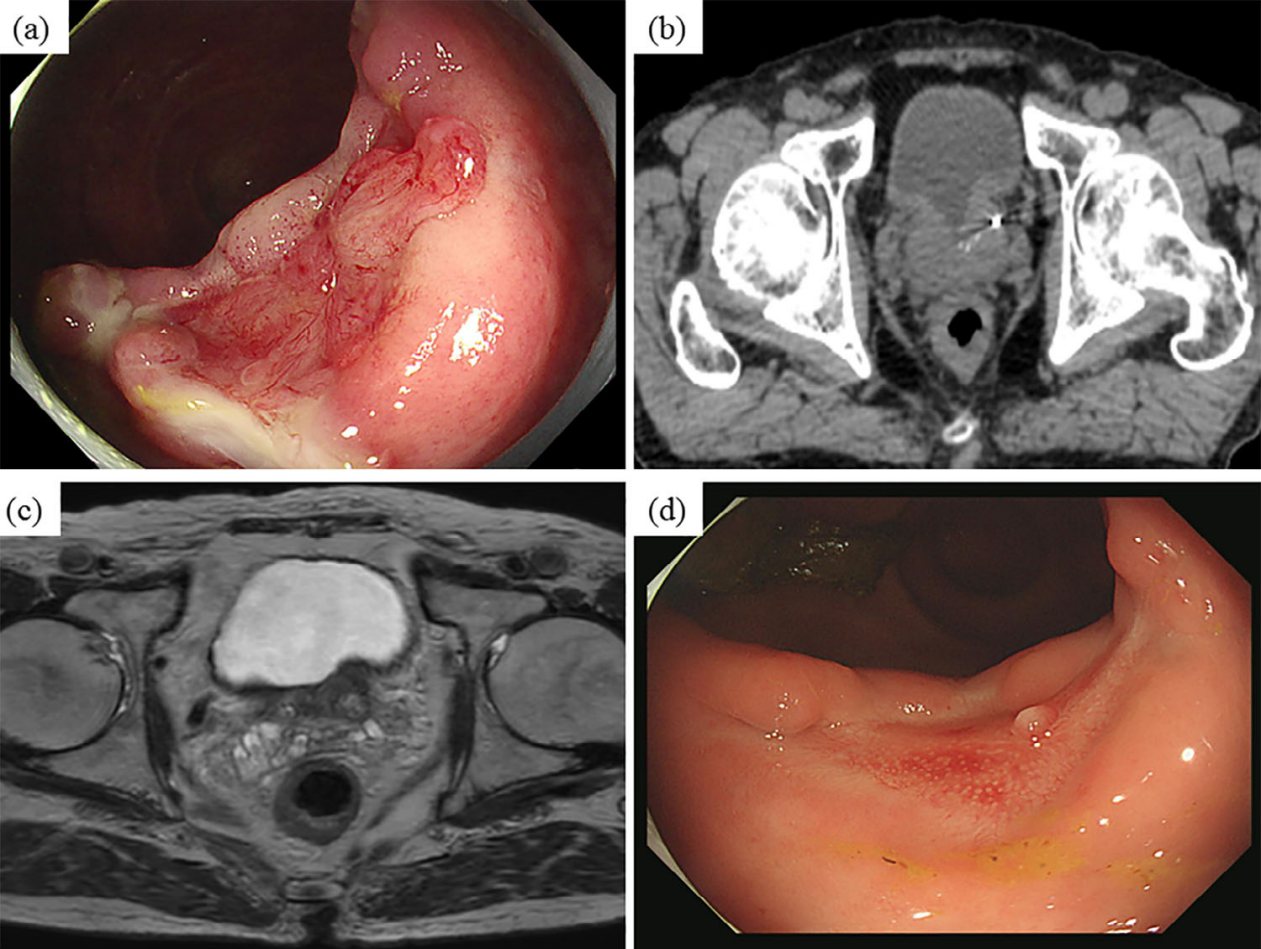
Colonoscopy (a), computed tomography (b), and magnetic resonance imaging (c) images obtained 2 months after the diagnosis indicating complete healing of the rectal ulcer. Scarring of the ulcer is observed during colonoscopy performed 6 months after hydrogel spacer insertion (d).
